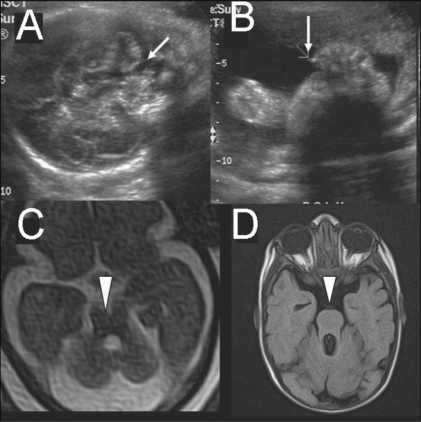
Single live gestation at 28 weeks. USG images (A,B) show inferior vermian agenesis (arrow in A) and postaxial polydactyly (arrow in B). T1W axial image (C) does not show the ‘molar tooth sign’ (arrowhead). Postnatal T1W axial MRI image at 4 months shows the ‘molar tooth sign’ (arrowhead), confirming the diagnosis of Joubert's syndrome. In this case, the mother noticed abnormal eye movements and failure of fixation of vision by the infant at 3 months of age
